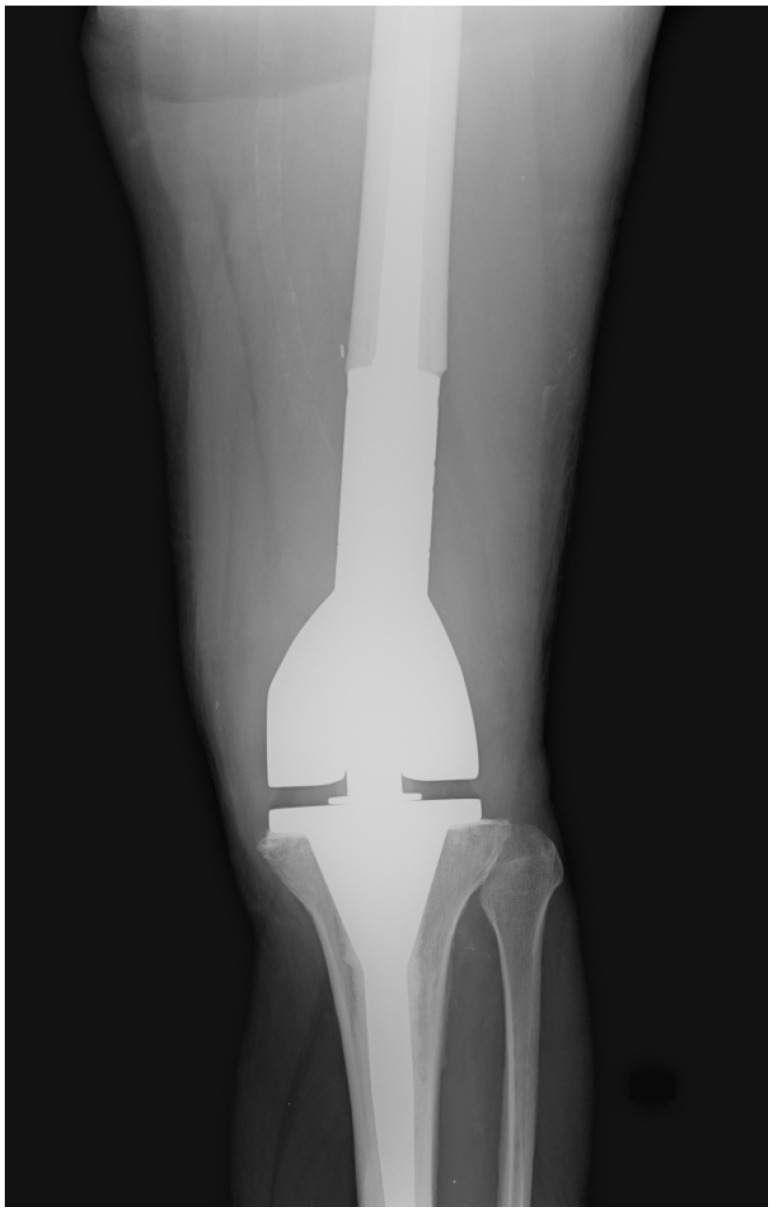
Post-operative X-ray of one of our patients treated with the implantation of a distal femur megaprosthesis.
